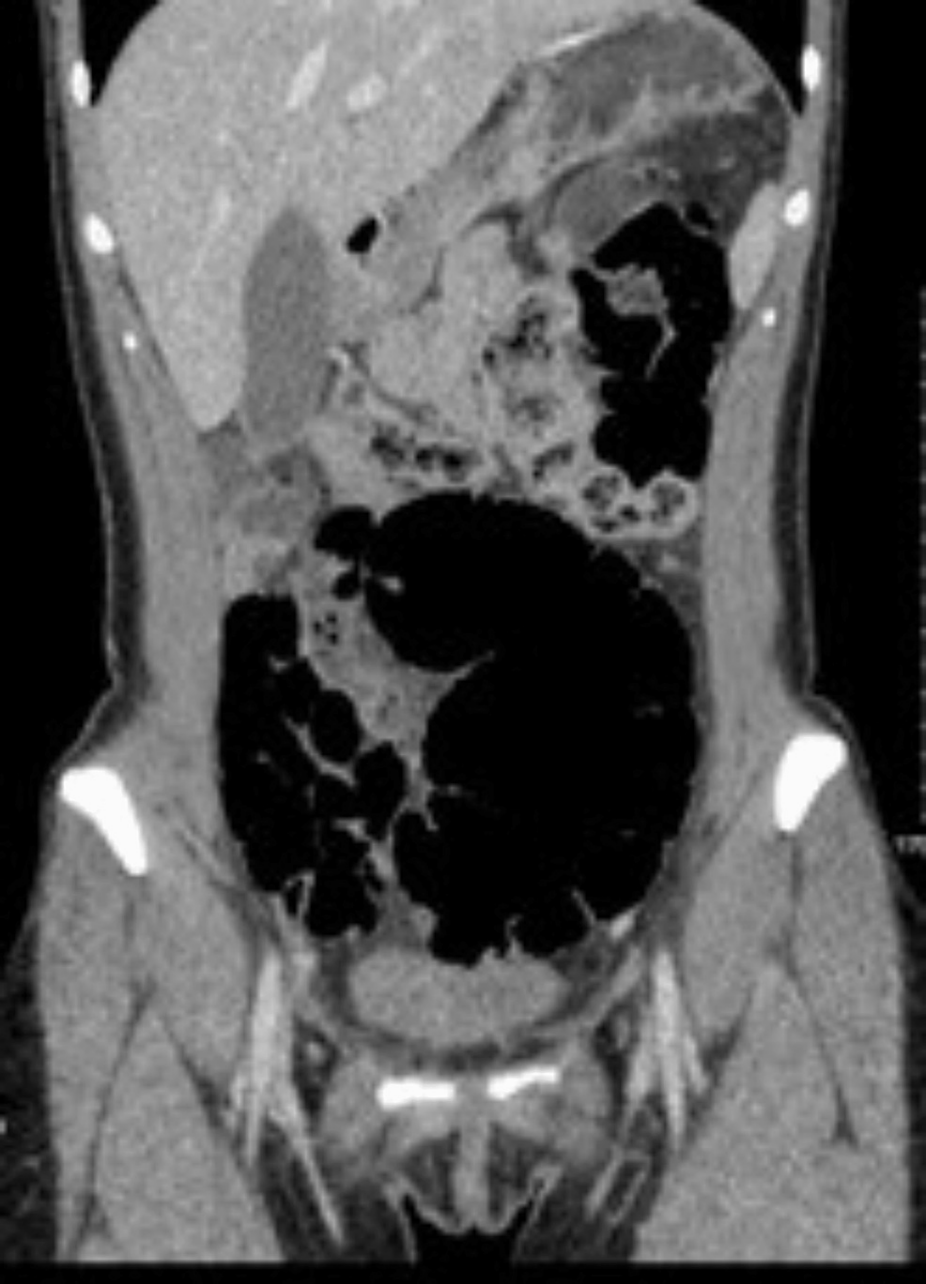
CT scan of abdomen and pelvis in sagittal view showing distended caecum, maximally 8.6cm
